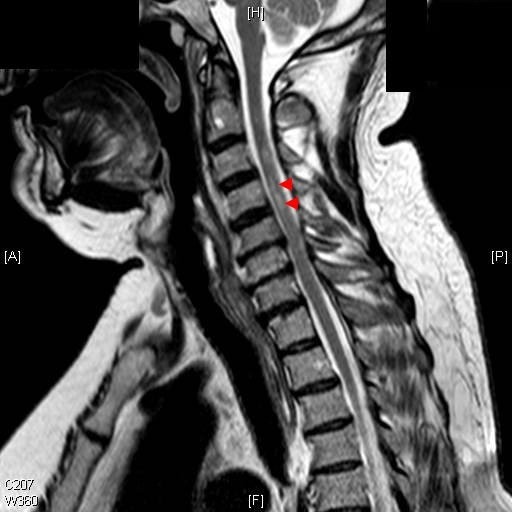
Axial T2-weighted magnetic resonance imaging (MRI) scan shows abnormally high signal intensity throughout the spinal cord at levels C3-C5. This is a common finding for regions of infarct.
